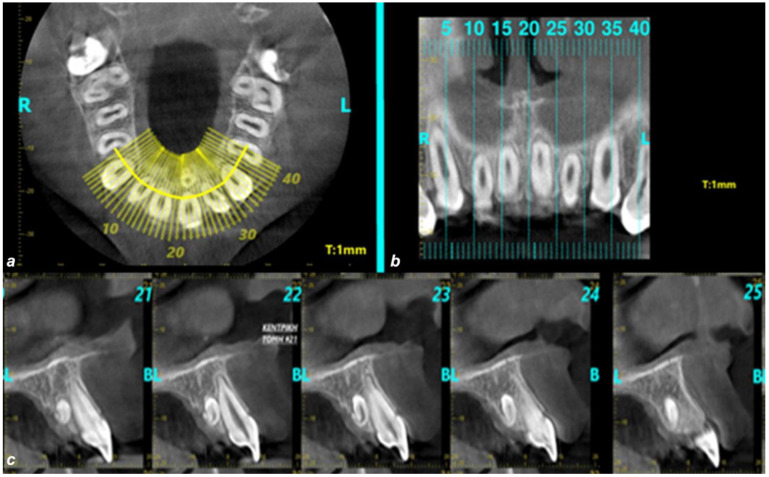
Sagittal images of the CBCT scanning (slice thickness 1 mm). The impacted supernumerary tooth (a) presents an inverted orientation (R: right, L: left.), (b) its root apex exhibits a pronounced palatal curvature due to limited space (R: right, L: left.), and (c) it is in contact with the palatal surface of the left central incisor without causing its resorption (B: buccal, L: lingual). The radiographic findings are indicative of an atypical supernumerary incisor.
